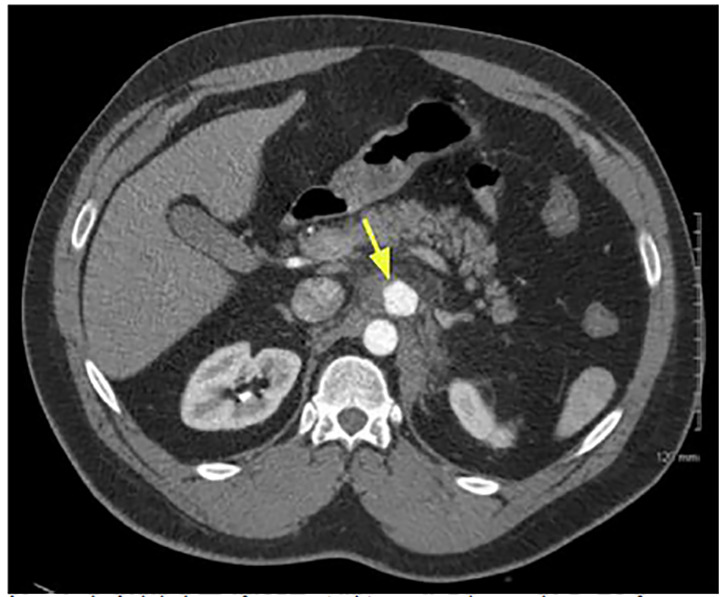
Axial view of computed tomography angiogram of abdomen and pelvis showing a proximal celiac artery aneurysm (arrow) with surrounding inflammation and trace hemorrhage.
